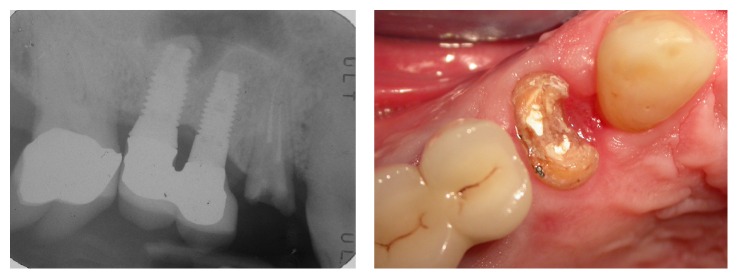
Case 1: clinical and radiographic evaluation before the extraction.
